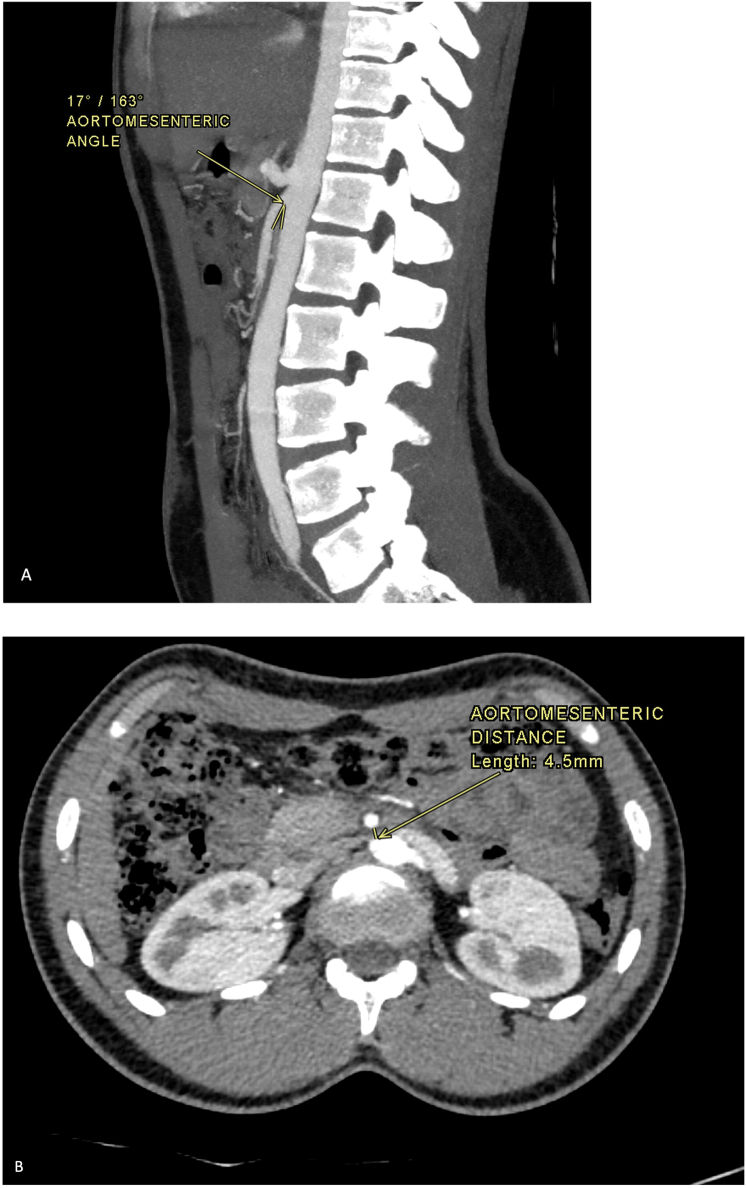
Contrast enhanced CT of the abdomen, sagittal image (A) and axial image (B). Acute angulation of the superior mesenteric artery was identified; The aortomesenteric angle and distance were both reduced measuring measured 17° and 4.5 mm respectively (measurements shown on A and B respectively).
